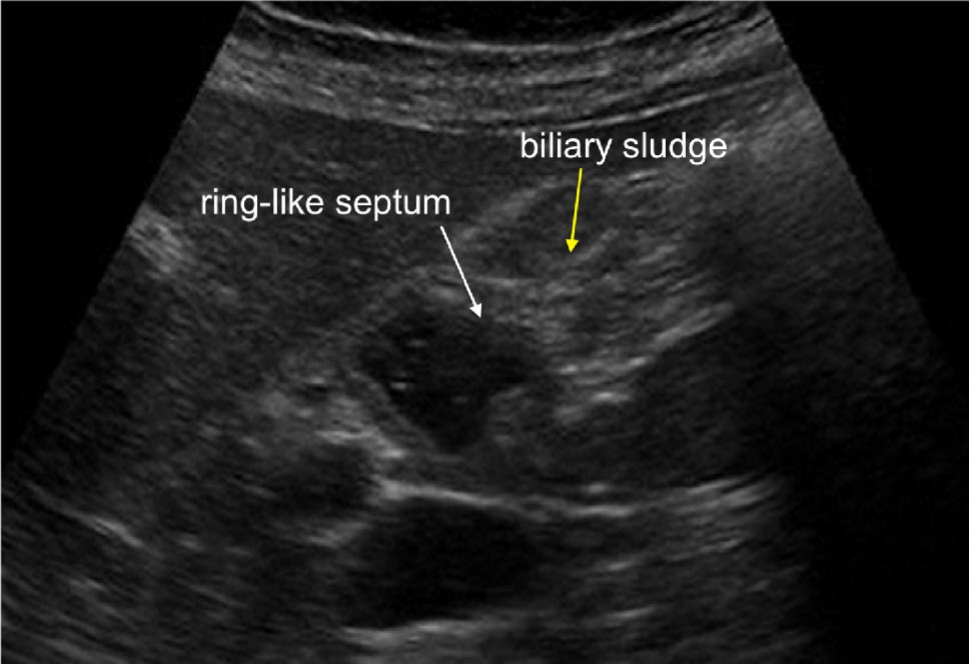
Sonography image depicts annular GA characterised by a ring-shaped (white arrow) circumferential thickening of the gallbladder wall, which usually involves the central part that divides the lumen of the gallbladder into two separate compartments and biliary sludge in the affected area (yellow arrow)
